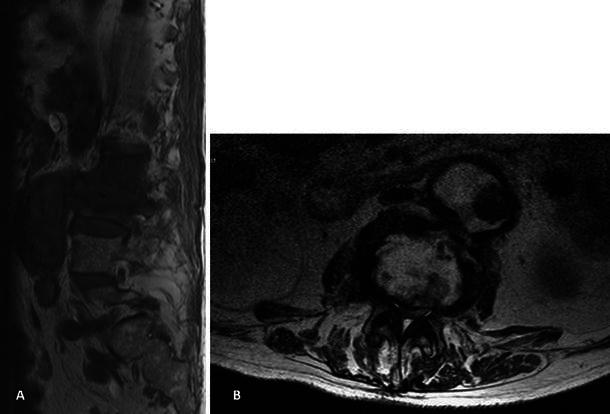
a MRI 9 months after endovascular abdominal aortic aneurysm repair showed the false aneurysm and its relation to L2–L3 vertebrae. b L2–L3 central stenosis
